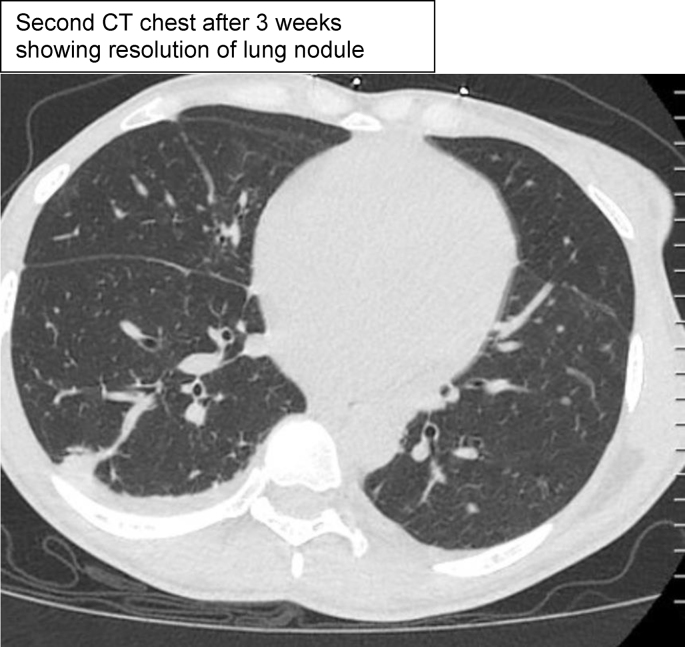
CT chest demonstrated multiple small nodular opacities in the right lower lobe and mediastinal lymphadenopathy. CT head and lumbar puncture were unremarkable. A Karius test was added due to her tenuous clinical status and returned positive for *PJP*, *Coccidioides posadasii*, *Histoplasma capsulatum*, and *Escherichia coli*. Later, *Histoplasma* urine antigen returned positive at a level of 0.5 (reference range <0.2ng/mL), *Coccidioides* serologies via enzyme immunoassay showed a + IgM, + IgG, and + CF, *Coccidioides* serum complement fixation titer returned at 1:16, *PJP* by DFA via BAL was positive, LDH was 305 (reference range 135–214 U/L), and Beta D glucan was >500 (reference range <60pg/mL). Aspergillus galactomannan antigen from the BAL (Platelia Aspergillus EIA kit from MiraVista Diagnostics) was 2.30 (reference range negative), but aspergillus serum antigen was negative, aspergillus was not isolated on cultures, and she did not have the clinical syndrome of aspergillosis. Cross-reactivity can be observed with aspergillus species. A Karius test was performed on the blood and ultimately detected high levels of *Pneumocystis jirovecii, Coccidioides posadasii,* and *Histoplasma capsulatum.*
